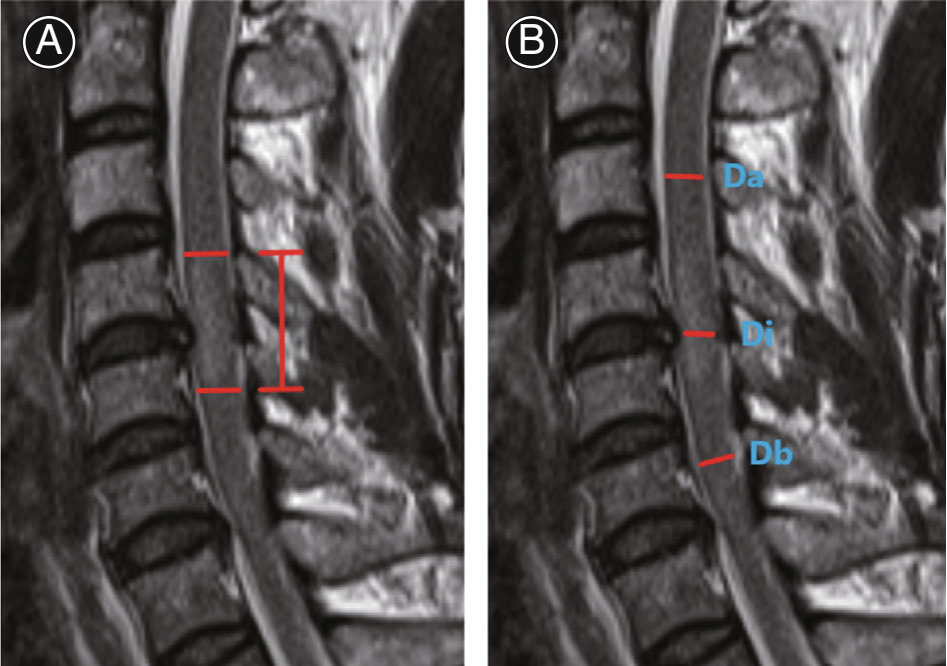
(A) Vertical length of high signal in spinal cord measured as the distance between the most cranial and most distal point of spinal cord edema in the mid‐sagittal plane on T2‐WI. (B) Based on the cord diameter at the level of maximum stenosis (Di), the normal level cord diameter closest to the level of stenosis (Da) and the cord diameter closest to the normal level at the caudal end of stenosis (Db) on T2‐weighted sagittal MR images. The maximum cord compression was also calculated as follows: Maximum spinal cord compression (%) = [1–2 × Di/(Da + Db)] × 100%
